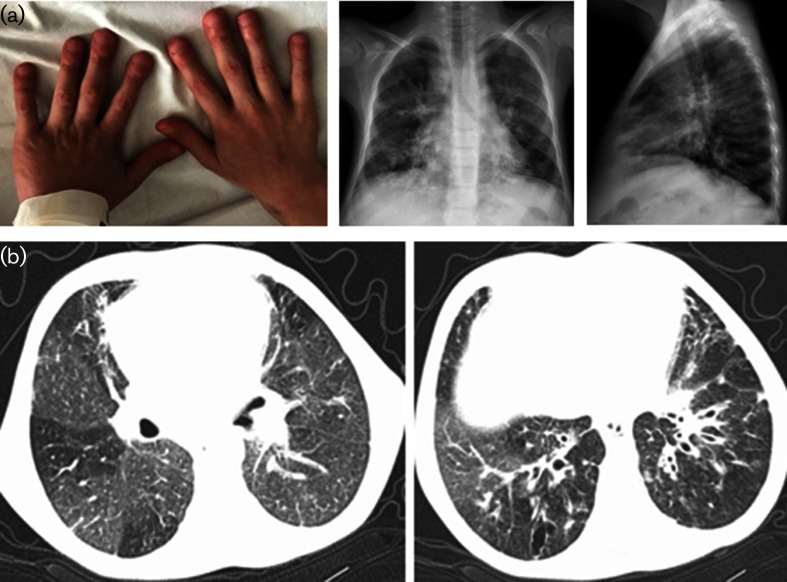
(a) Clubbing and chest X-ray of Patient 1 (at 9 years of age): bilateral heterogeneous ill-defined lung infiltrations with peribronchial thickening. Air trapping on lateral chest X-ray. Mediastinal widening due to lymphadenomegaly. (b) Thorax CT of Patient 1 (at 9 years of age): bilateral mosaic perfusion defect with basal bronchial wall thickening and bronchiectasis.
